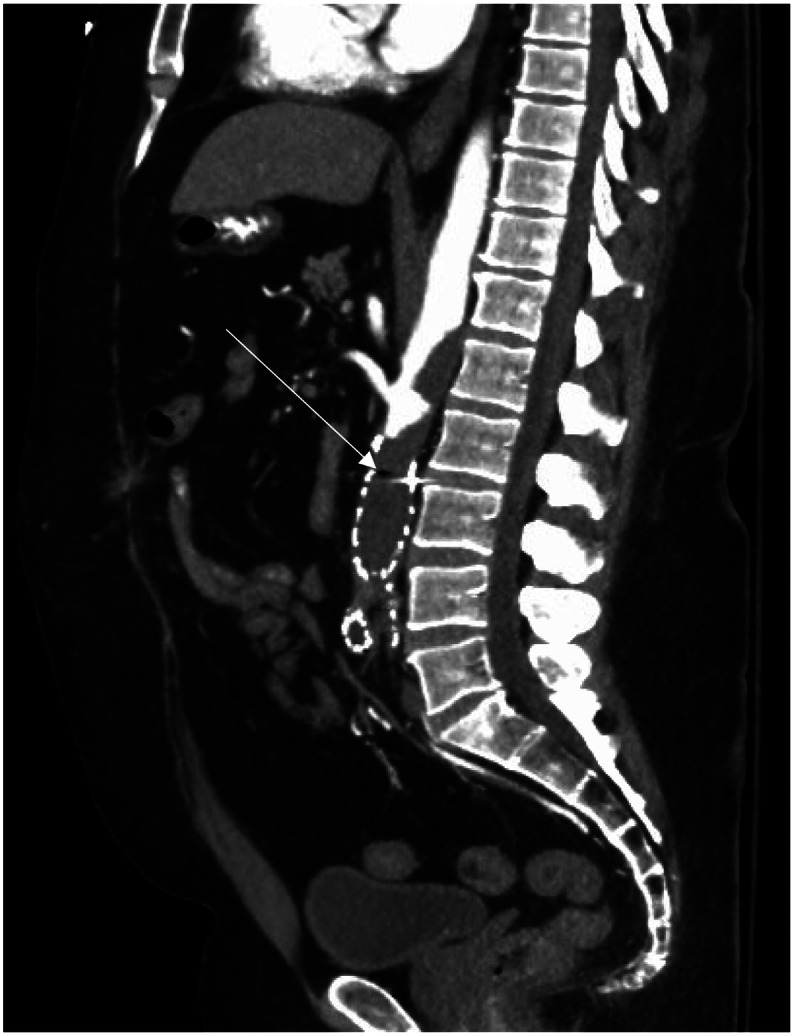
Computed tomography angiogram abdomen in sagittal plane showing degree of clot burden within aortic stent graft (thin arrow).
